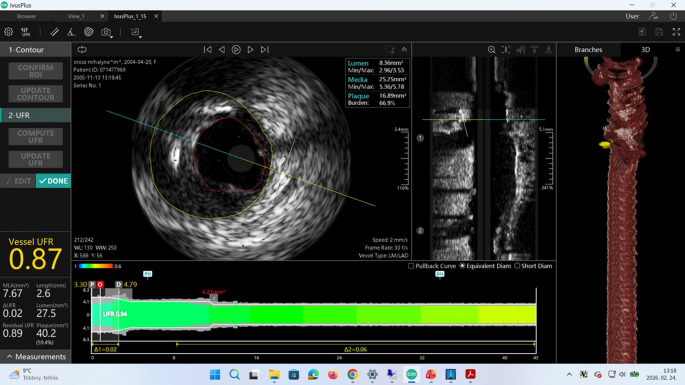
Ultrasonic flow ratio (UFR).
